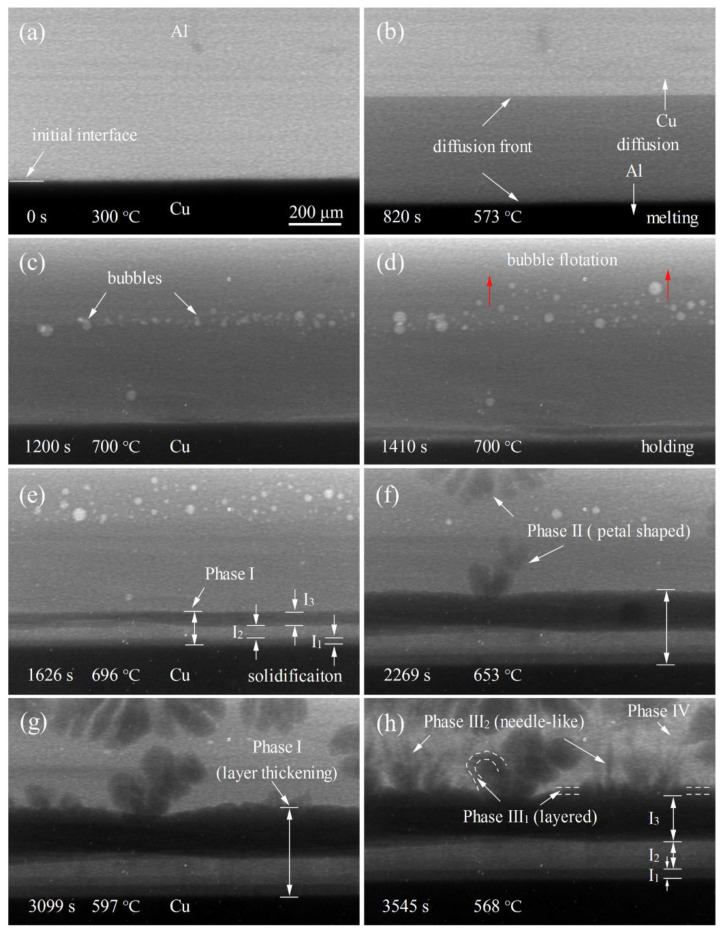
Sequence of in situ radiographs showing the interfacial diffusion and microstructure evolution in the Al/Cu sample: (a–d) the melting and holding process (*t* = 0 s is assigned to the onset of image collection at 300 °C), (e–h) the solidification process after holding at 700 °C for 5 min.
